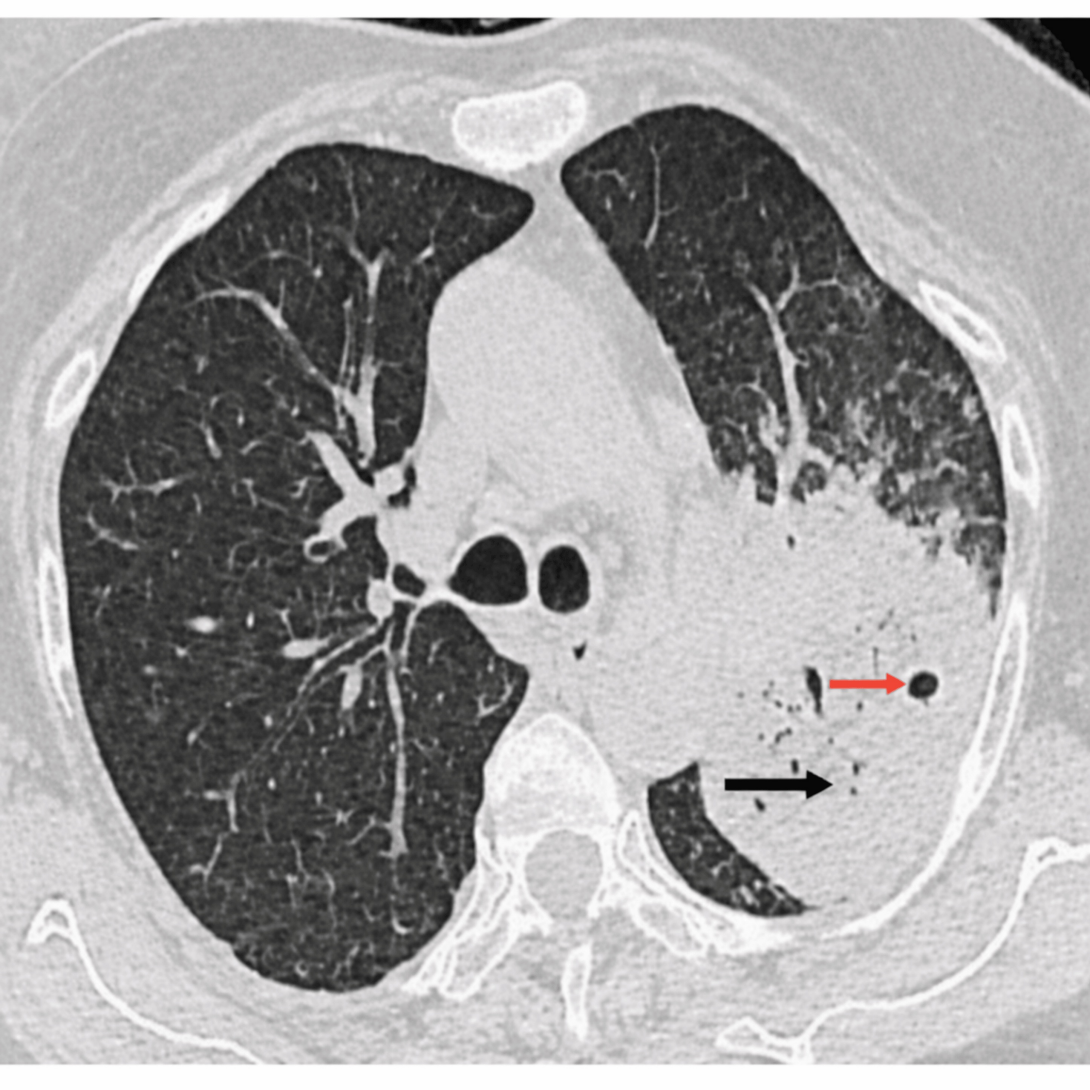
High-resolution computed tomography of the chest showing consolidation (black arrow) with cavitation (red arrow) involving the left upper lobe posterior segment and lingula
